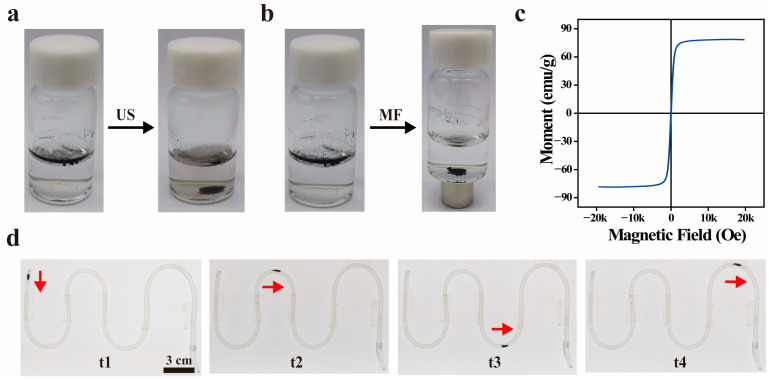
MF/US-responsive properties of FMB. (a) Photographs of FMB before and after ultrasound irradiation. (b) Photographs of FMB before and after the action of MF. (c) Vibrating sample magnetometer (VSM) spectra of FMB. (d) Photographs of FMB migrating to the target position in the catheter under the action of a permanent magnet. t1, t2, t3, and t4 represent different time points. The red arrow indicates the moving direction of FMB.
